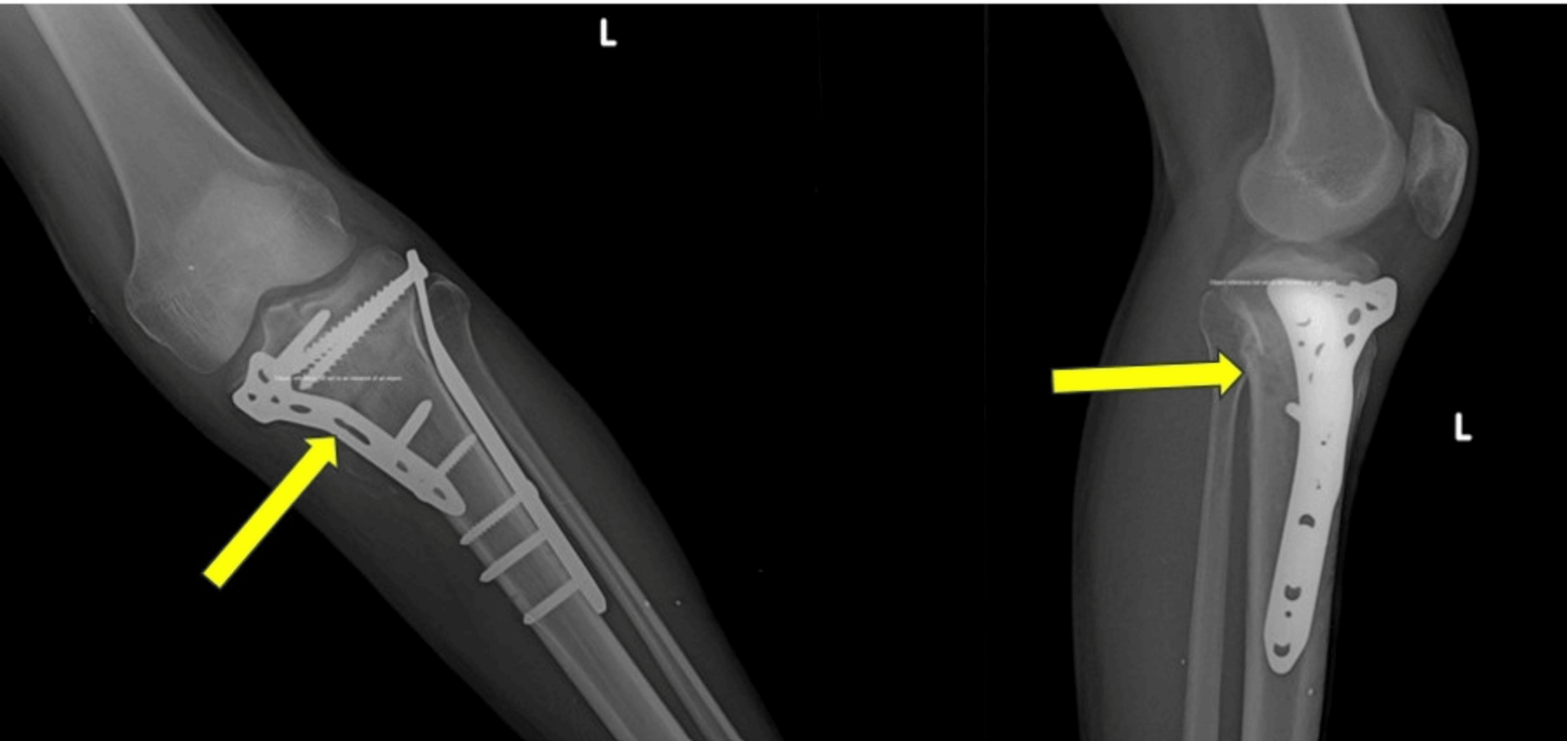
Final follow-up radiographs at six months demonstrating fracture union and maintained alignment
